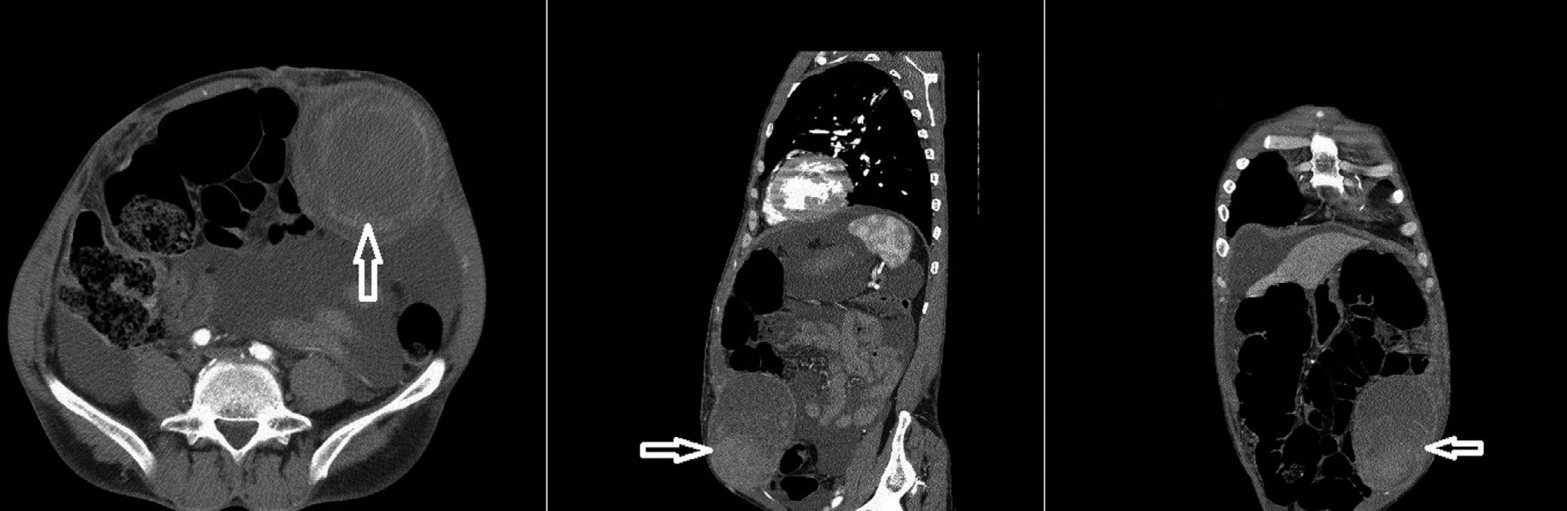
Contrast‐enhanced computed tomography (CT) section of the abdomen and pelvic demonstrated an oval circumscribed structure in the left rectus muscle with mixed attenuation. (arrows)
